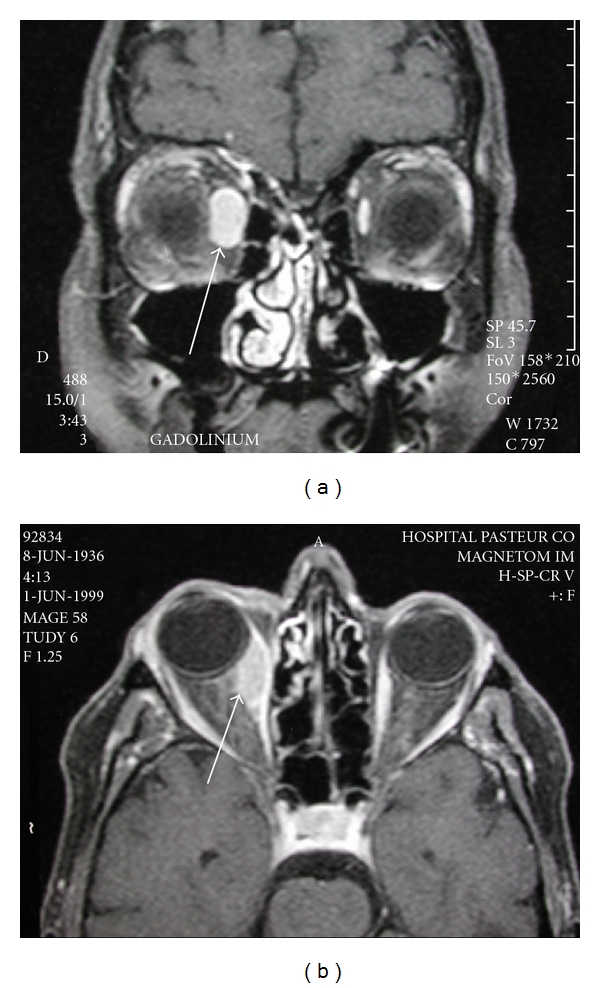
High signal intensity on MRI images in an orbital myositis related to Lyme disease.
